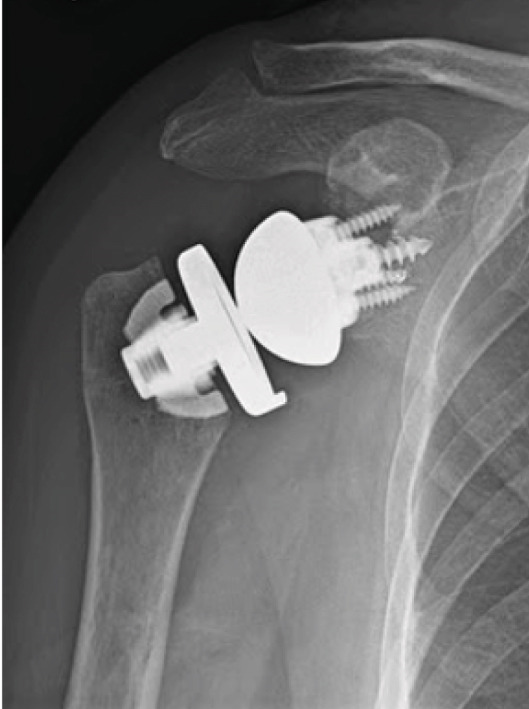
Shoulder radiograph demonstrating a stemless reverse TSA with no signs of loosening at 1‐year postoperative appointment (April 12, 2023).
